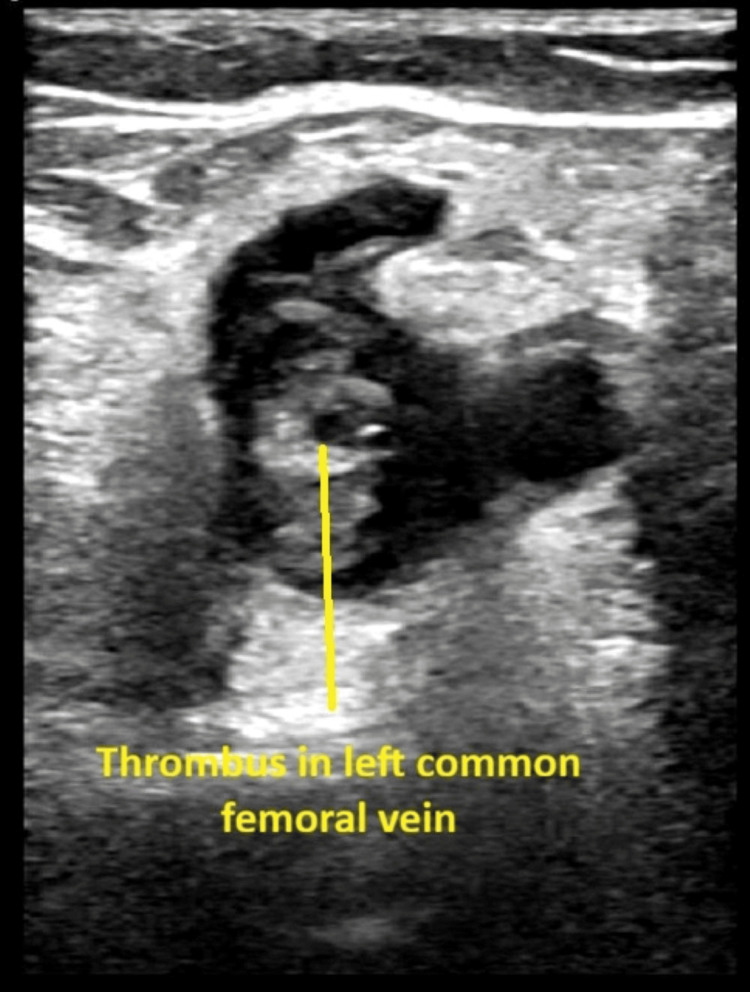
Enlarged section of the CT image showing thrombus in the left common femoral vein
